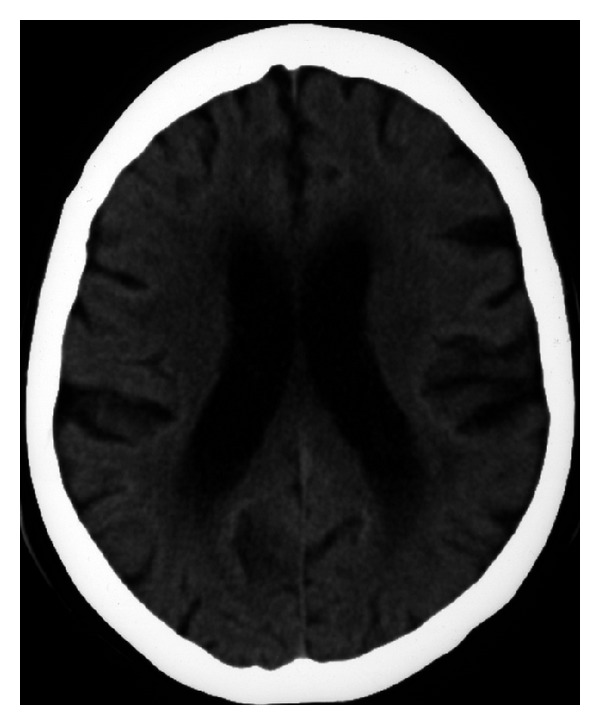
Brain CT-scan performed during follow-up in 2008, showing periventricular white matter damage (left-right was inverted as radiological convention).
